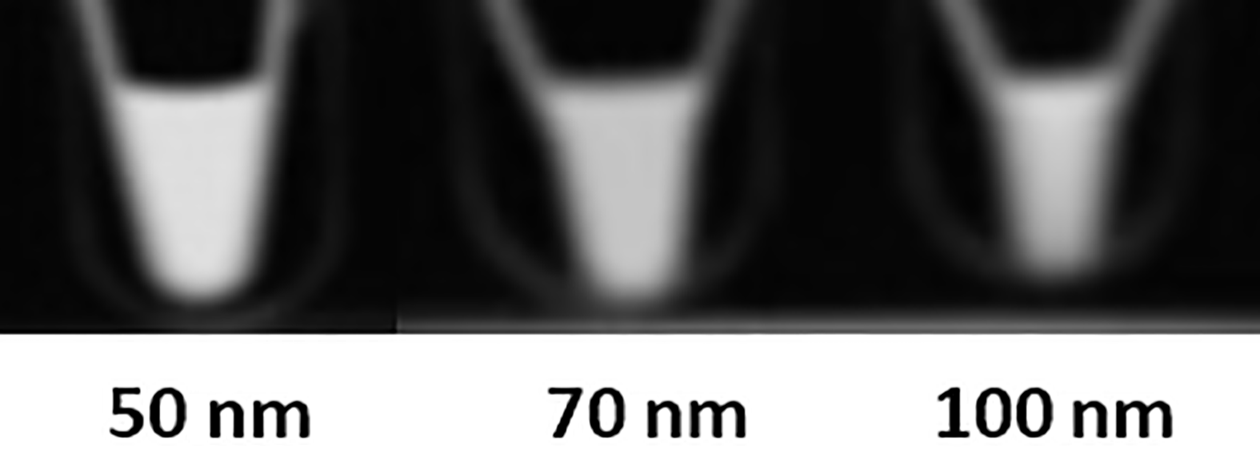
CT images of Au-CPMV particles of different sizes suspended in DD water at, the gold concentration is 200 μg of gold suspended in 200 μl aqueous solution for each sample. The average CT values from three scans for each sample were 183 HU, 133 HU, and 115 HU for the 50 nm, 70 nm, and 100 nm, respectively.
